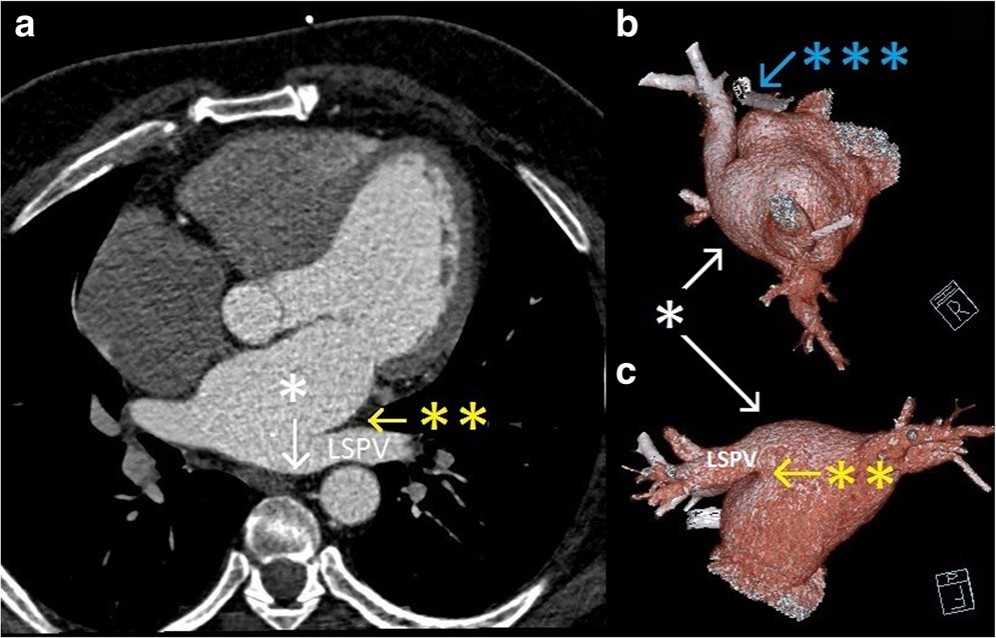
Angio-CT scan of the left atrium (A) and 3D reconstructions (B and C). Single asterisk denotes a bulging roof of the left atrium (white); double asterisks, a grove after epicardial box ablation (yellow); and triple asterisks, LAA AtriClip (blue)
